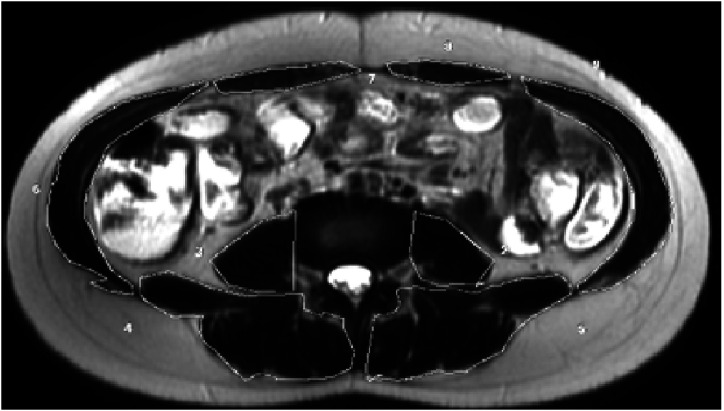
Skeletal muscle area was calculated at the same level with psoas muscle at L4 vertebrae on the axial T2-weighted images.
